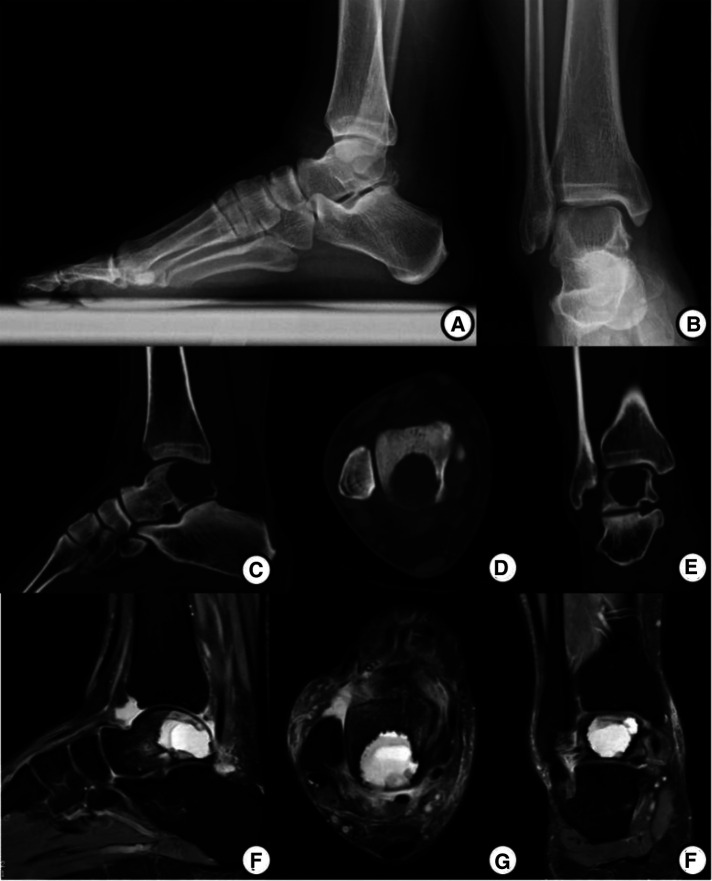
Preoperative imagological data. (A,B) AP and Lateral x-ray before operation show a well-demarcated and lytic lesion in the posterior talus. (C–E) Preoperative CT images show a lesion. (F–H) Preoperative MRI images show a lesion with no cortical and articular surface breakthrough, measuring 2.5*2.2*2.2 cm.
